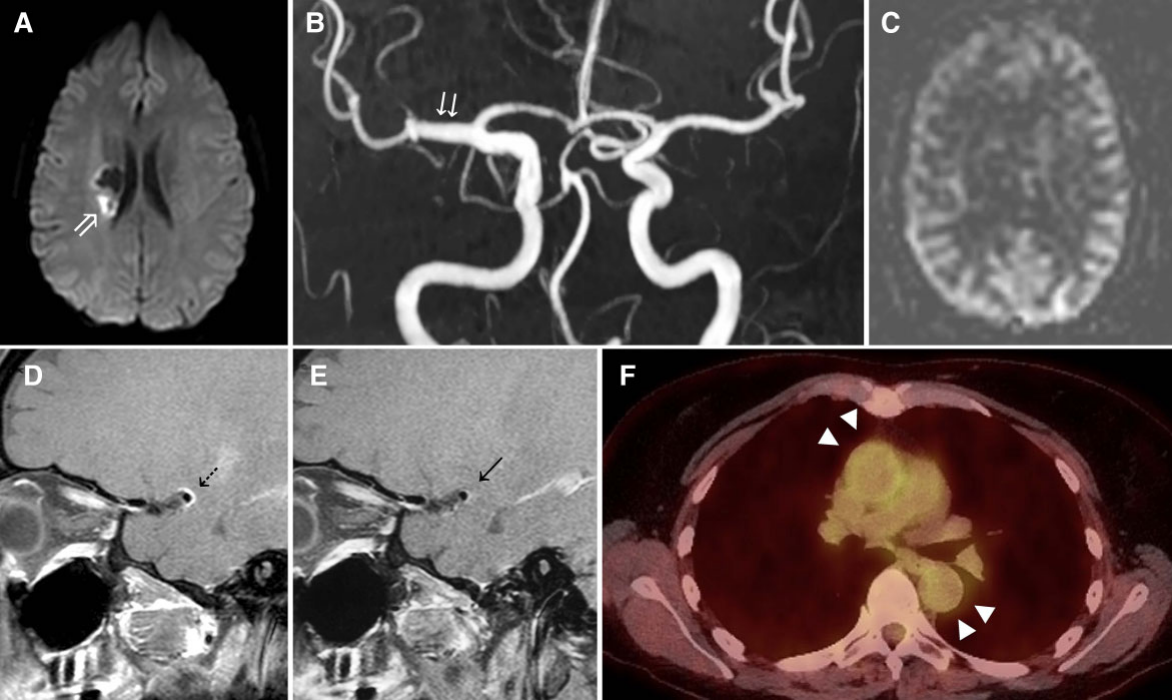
Intracranial Takayasu (Patient 10); Intracranial Takayasu arteritis: Axial b1000 DWI image (A) demonstrates an acute right striatocapsular infarct, with fusiform dilatation of the M1 segment of the right MCA on time-of-flight MRA (B). ASL perfusion-weighted image (C) shows the reduced signal intensity of labelled spins in the right peri Sylvian regions, which normalized on follow-up imaging (not shown). Axial-fused (F) ^18^FDG PET-CT image demonstrates concentric and increased radiotracer uptake within the wall of the ascending and proximal descending thoracic aorta (solid black arrow). Baseline sagittal VWI (D) shows concentric thickening and enhancement of the vessel wall of the right MCA (dotted black arrow), with interval improvement on subsequent VWI following interim treatment (E).
